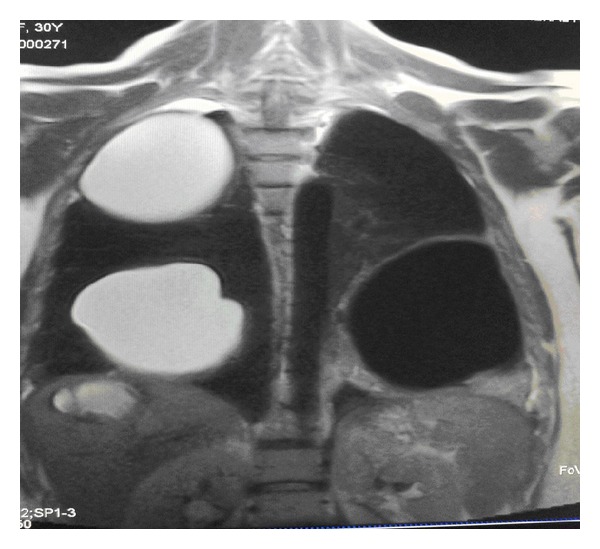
MRI of the thorax showed ruptured hydatid cyst of the left lung and multiple other intact hydatid cysts of the right lung and liver.
